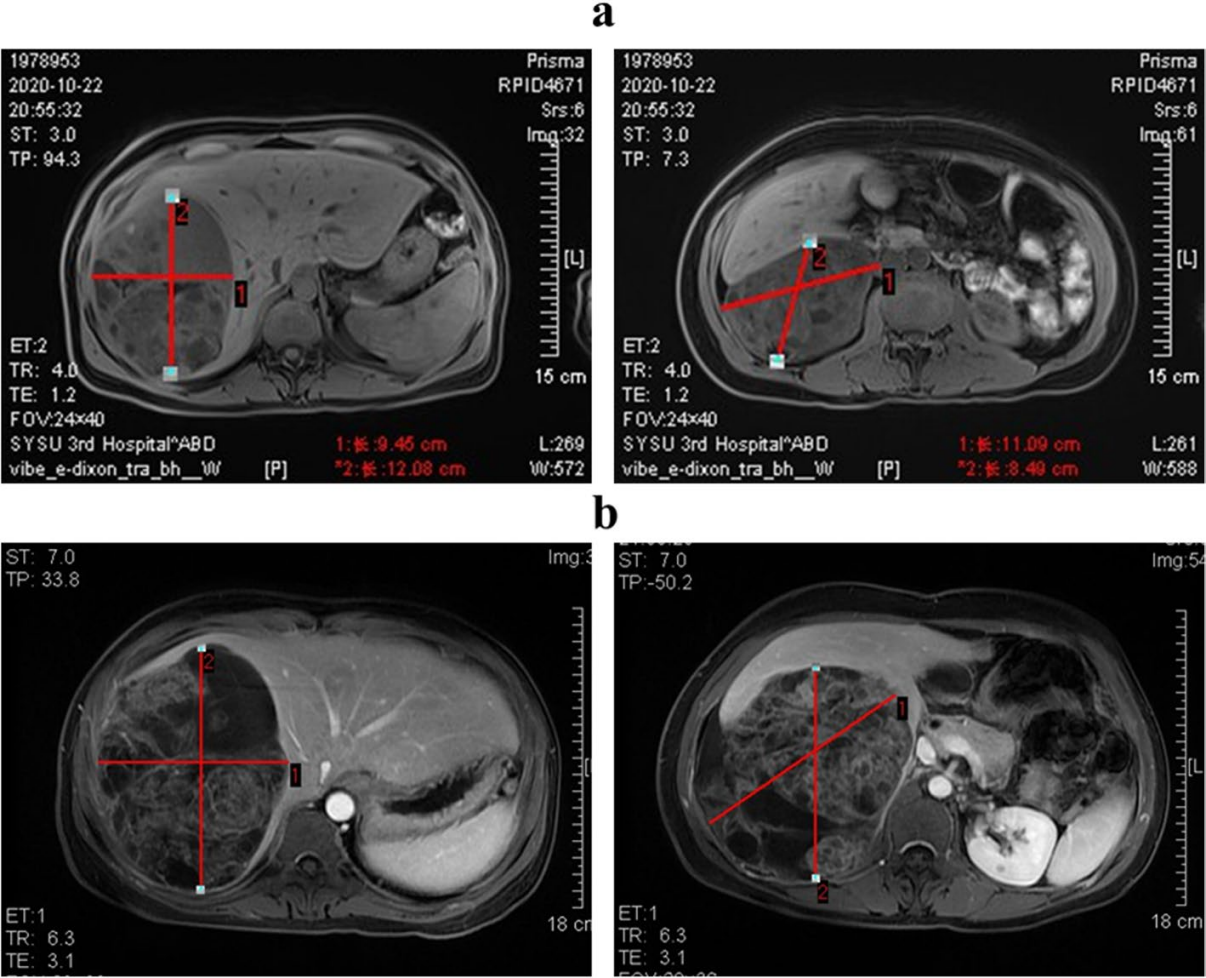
a MRI examination of the upper abdomen on October 22, 2020. b MRI examination of the upper abdomen on January 29, 2021 showed that the teratoma of the liver was alive and slightly bigger than before. Note: left picture represents the largest section of the mass in S VII-VIII of liver while the right one shows the largest section of the mass in S V-V
